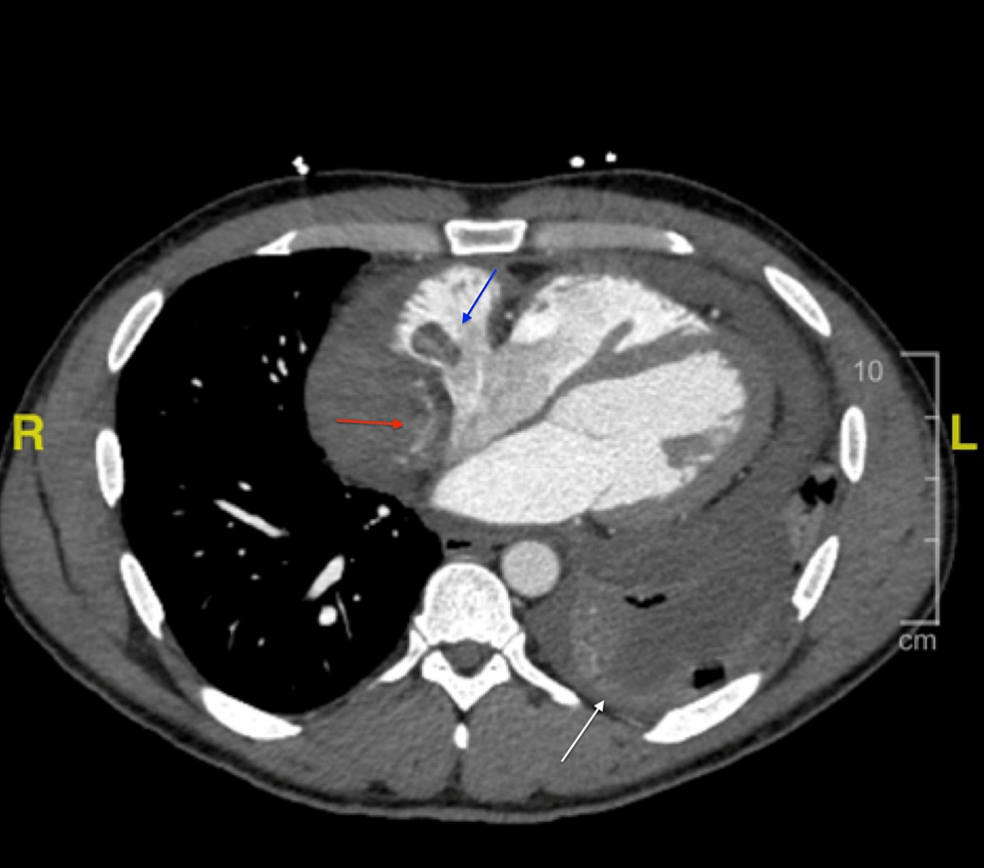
CT chest angiogram demonstrating complex hyperattenuating pericardial effusion, with contrast seen adjacent to the right (red arrow). A focal filling defect was also appreciated within the right atrium, measuring 1.9 cm (blue arrow). A moderate-sized left-sided pleural effusion was also noted (white arrow).
